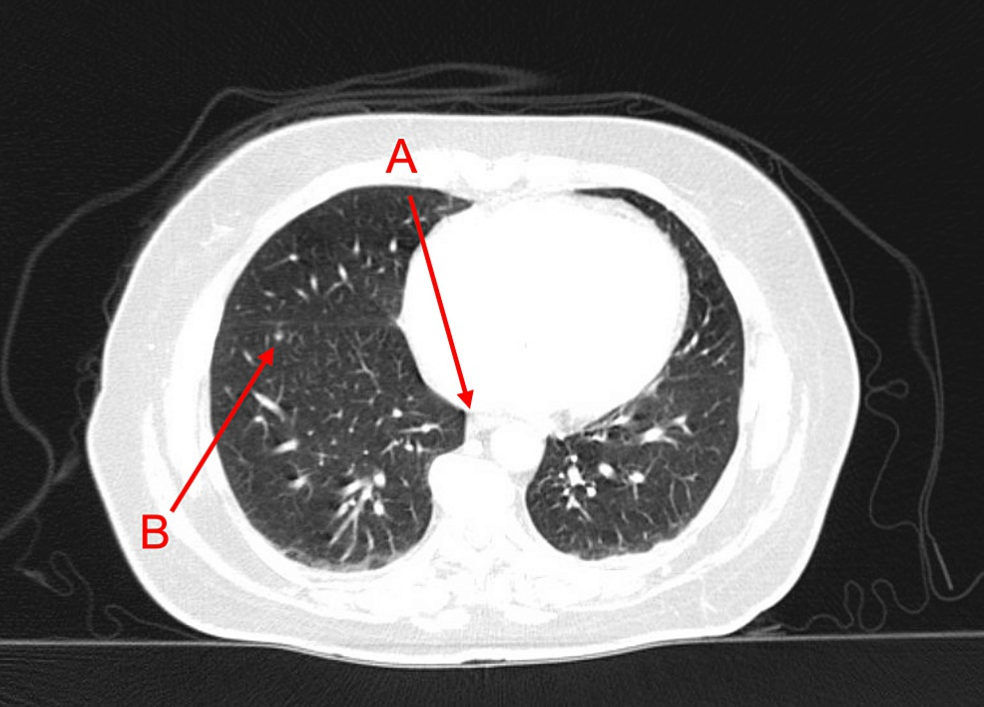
Computed tomography of the chest with contrast Computed tomography of the chest with contrast showing prominent mediastinal hilar lymph nodes (arrow A) and a right lower lobe pulmonary nodule measuring 4-5 mm (arrow B).
